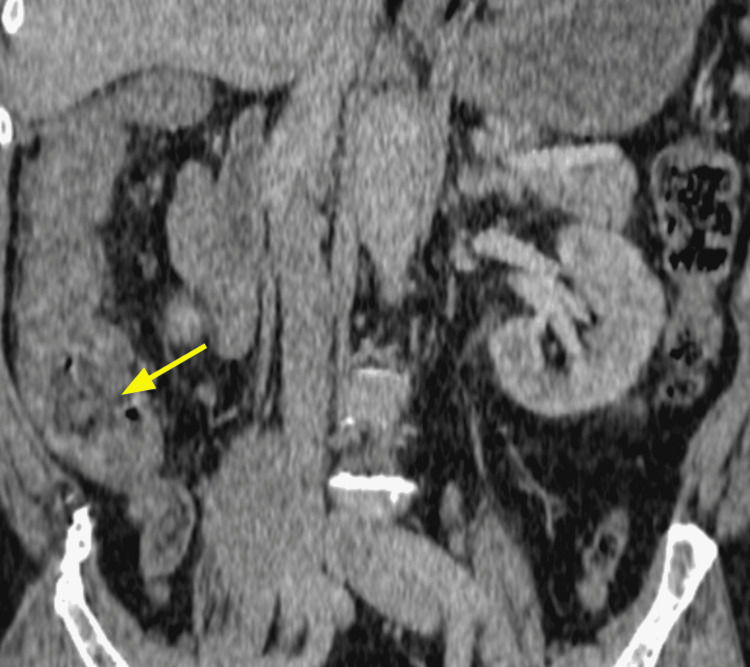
Contrast-enhanced CT for Case 2 (coronal plane) demonstrating large right bowel and thickening of the caecum wall with infiltration of the pericolic fat (arrow)
